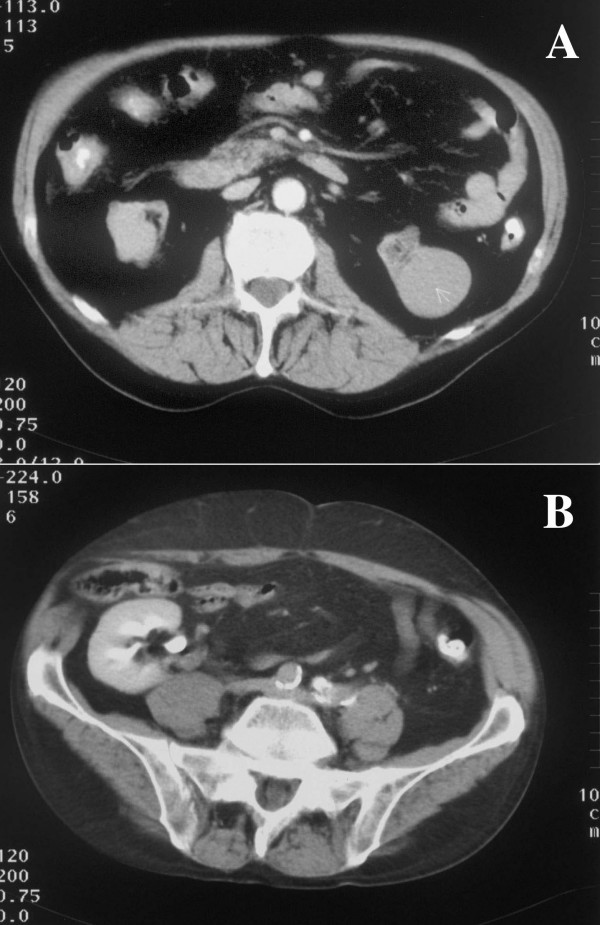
CT in the supine position. Panel A: The wall of the ascending colon was interpreted as 'thickened'. Panel B: CT at the level of the cecum showing the renal transplant kidney functioning well. The kidney is adjacent to the ascending colon and cecum.
